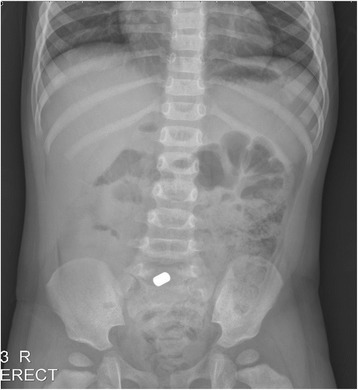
Abdomen radiography showed metallic foreign body in the abdominal cavity
